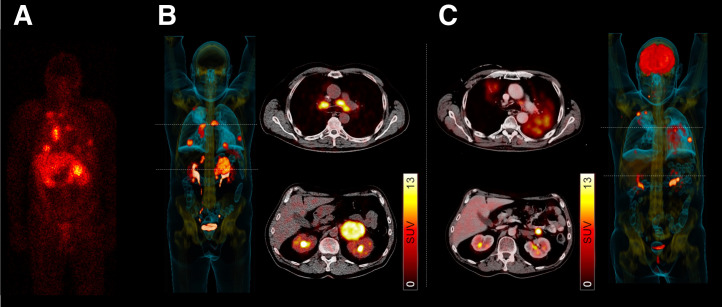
Synergistic effects of radioimmunotherapy and CXCR4-targeted RLT in patient with heavily pretreated diffuse large B-cell lymphoma. (A) Pretherapeutic scintigraphy 24 h after ^177^Lu-pentixafor injection revealed multiple disease sites, allowing for calculations of absorbed doses. (B) Baseline maximum-intensity projection and transaxial ^68^Ga-pentixafor PET/CT showed multiple CXCR4-expressing mediastinal and abdominal lesions. (C) ^90^Y-ibritumomab tiuxetan combined with ^90^Y-pentixather was initiated. On ^18^F-FDG PET/CT 4 mo later, sites of disease were smaller, indicating PR. (Modified from (63).)
